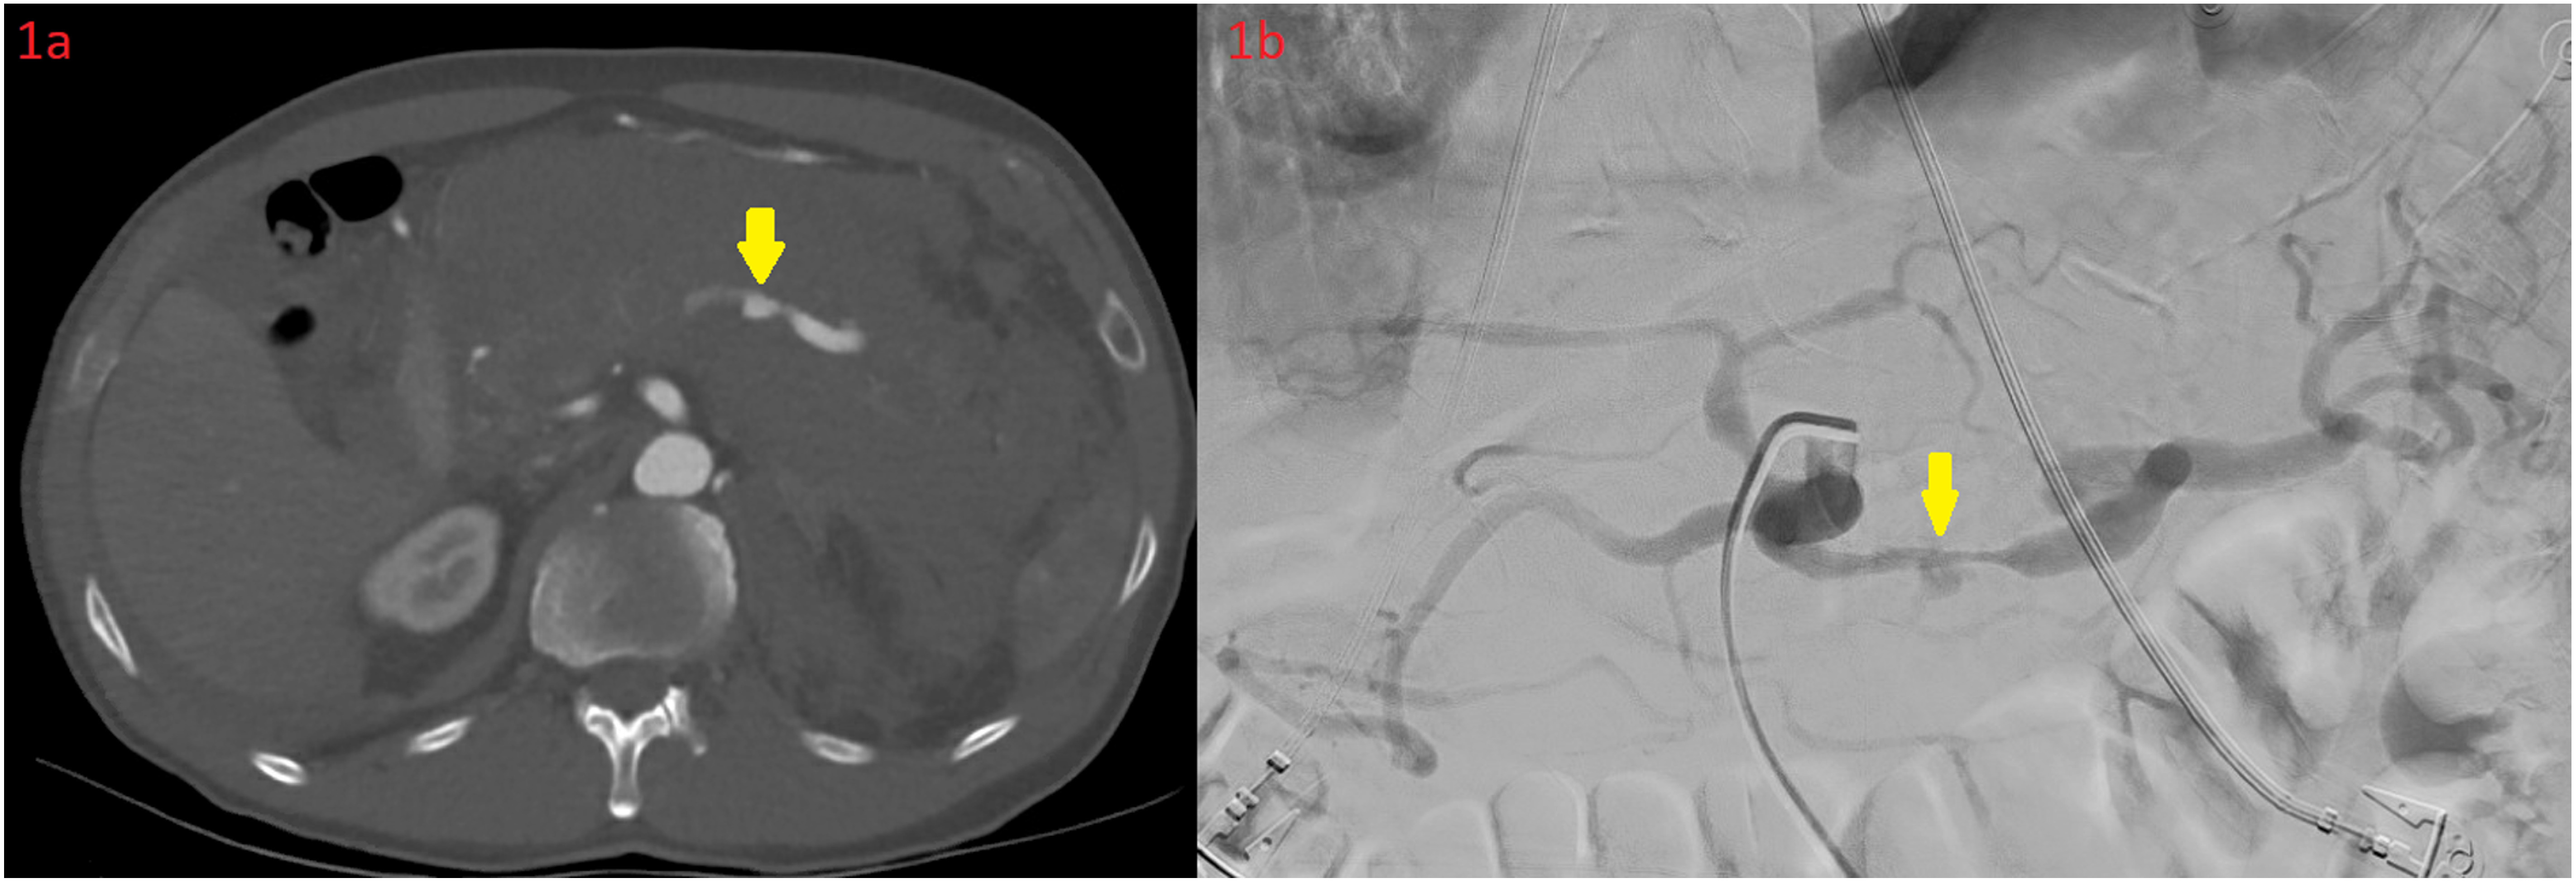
Fig. 1 (a) Computed tomography angiogram demonstrating splenic artery pseudoaneurysm surrounded by retroperitoneal haemorrhage. (b) Angiography demonstrating proximal splenic artery pseudoaneurysm.
